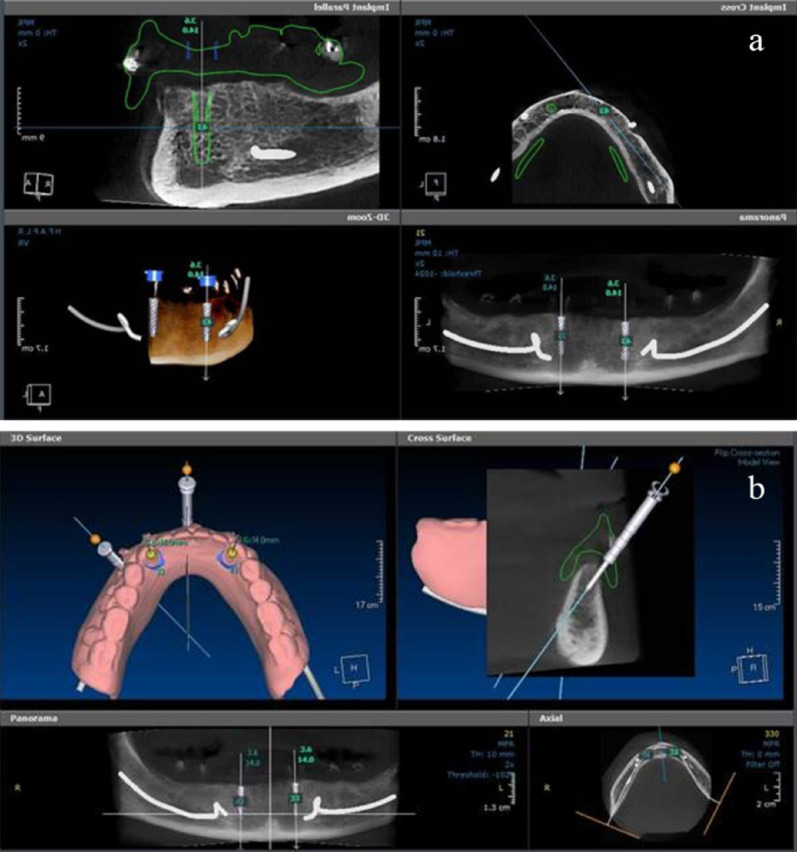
a, Preoperative CBCT-based digital planning of mandibular implant placement. Multiplanar views demonstrate implant angulation, depth, and relation to anatomical landmarks. b, Virtual guide design is shown to ensure prosthetically driven implant positioning.
